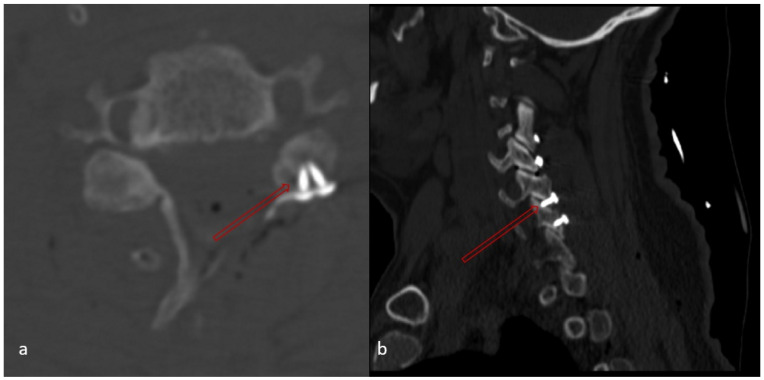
Facet joint disturbance at the C5–6 level: (a) red arrow shows screws on lateral mass disturbing facet joint on axial CT; (b) red arrow shows facet joint disturbance on sagittal CT.
